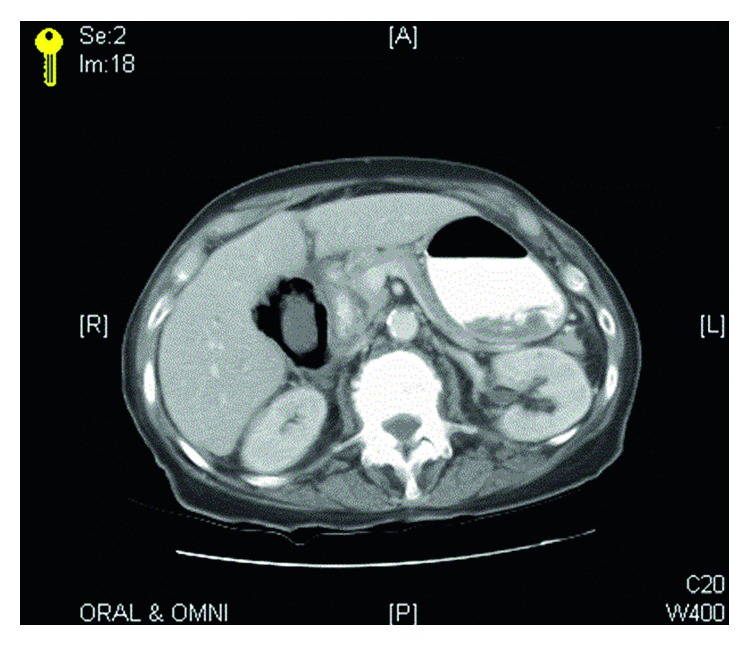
CT scan. Extensive intramural gas with a thickened gallbladder wall surrounded by marked inflammatory changes.
